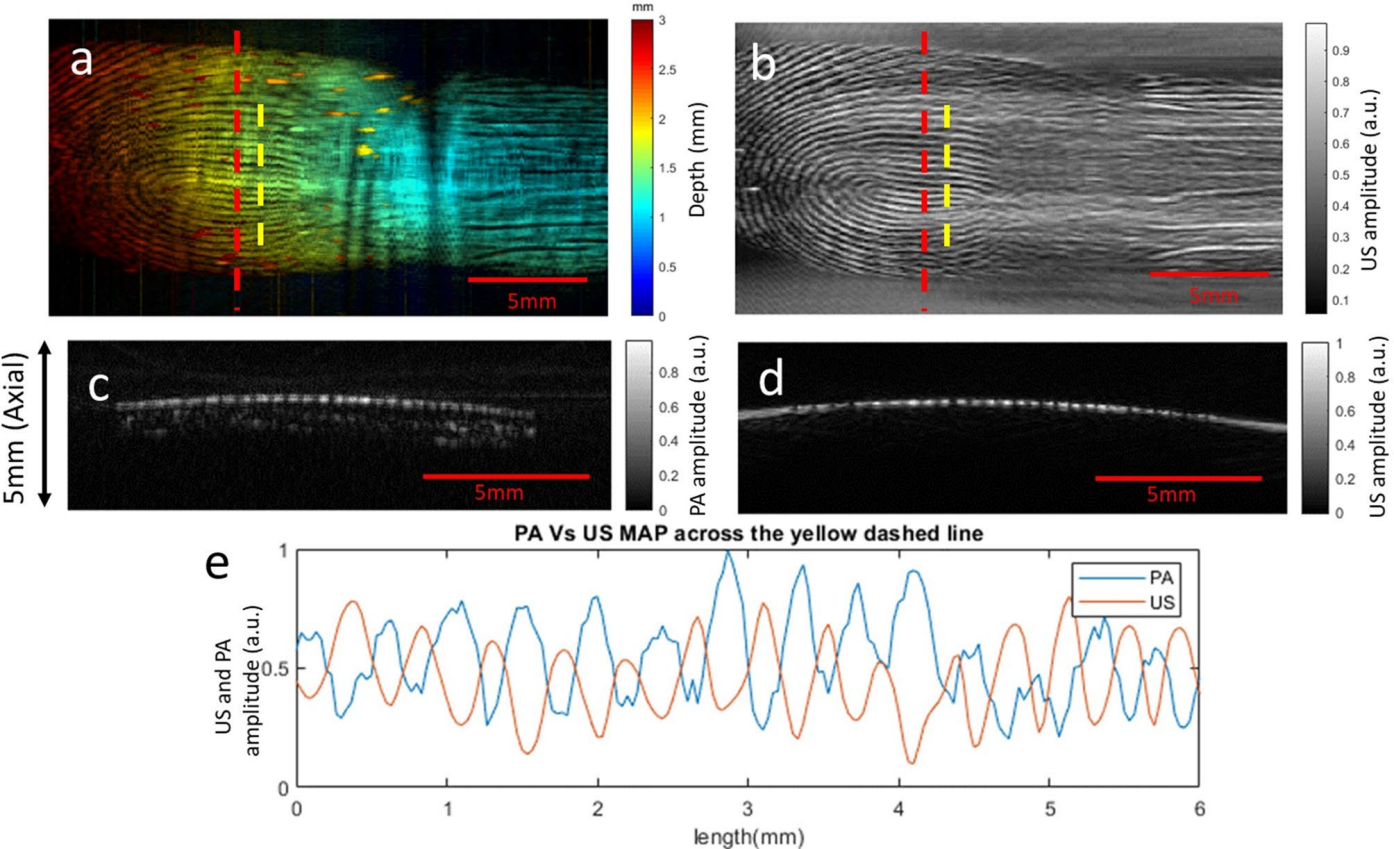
Photoacoustic and ultrasound images of a dry finger. (a) Depth-encoded photoacoustic image. (b) Gray-scale ultrasound image. (c) Cross-sectional PAT image across the red dashed line in (a). (d) Cross-sectional US image across the red dashed line in (c). (e) Intensity profiles of the ultrasound and photoacoustic images across the yellow dashed lines in both (a) and (b).
